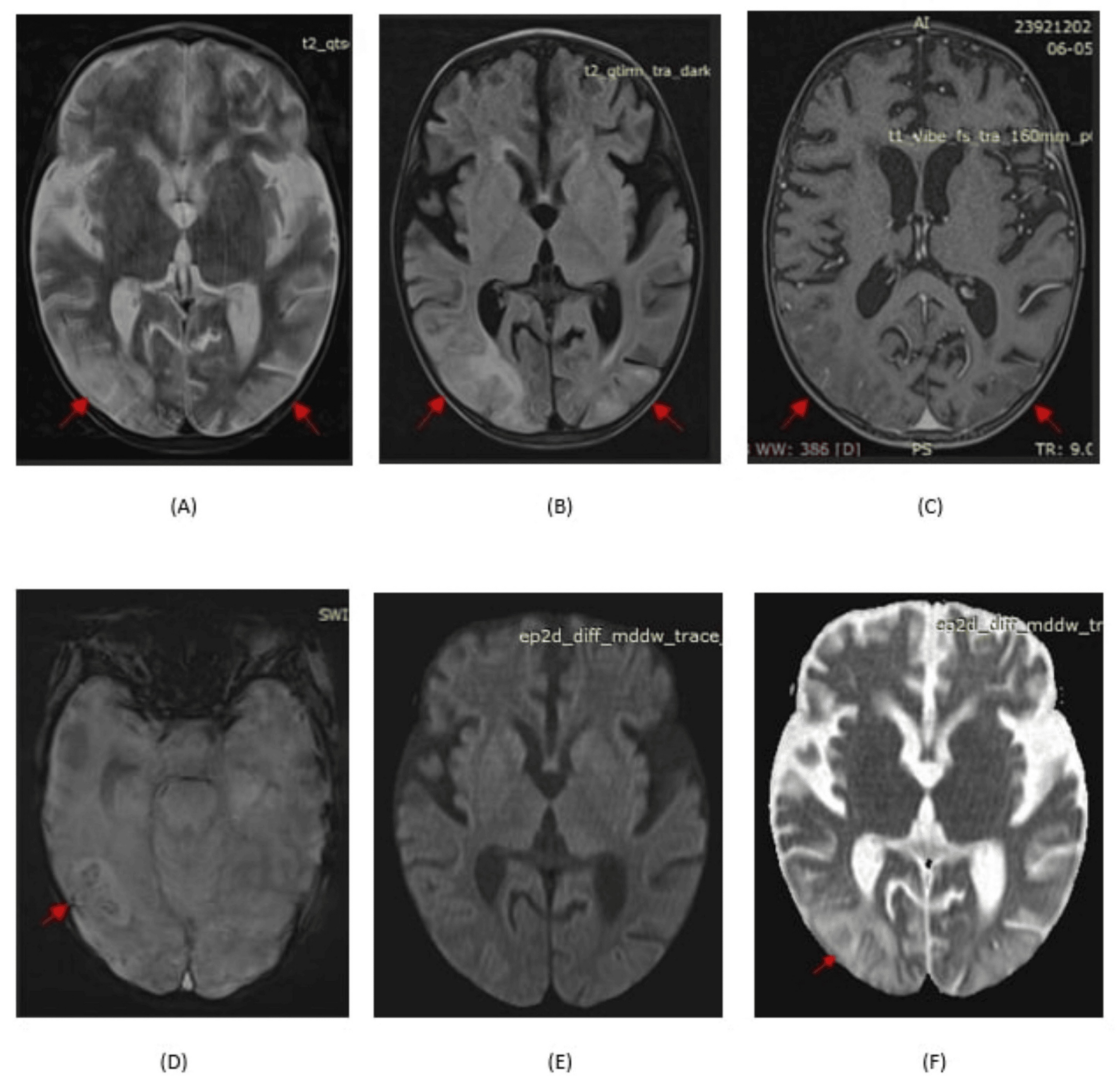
MRI brain showing bilateral parieto-occipital hyperintensities on T2 and FLAIR (A, B), hypointensities on T1 (C), microhemorrhages on SWI (D), no diffusion restriction on DWI (E), and facilitated diffusion on ADC (F), findings consistent with vasogenic edema ADC: apparent diffusion coefficient; FLAIR: fluid-attenuated inversion recovery; DWI: diffusion-weighted imaging; SWI: susceptibility-weighted imaging
